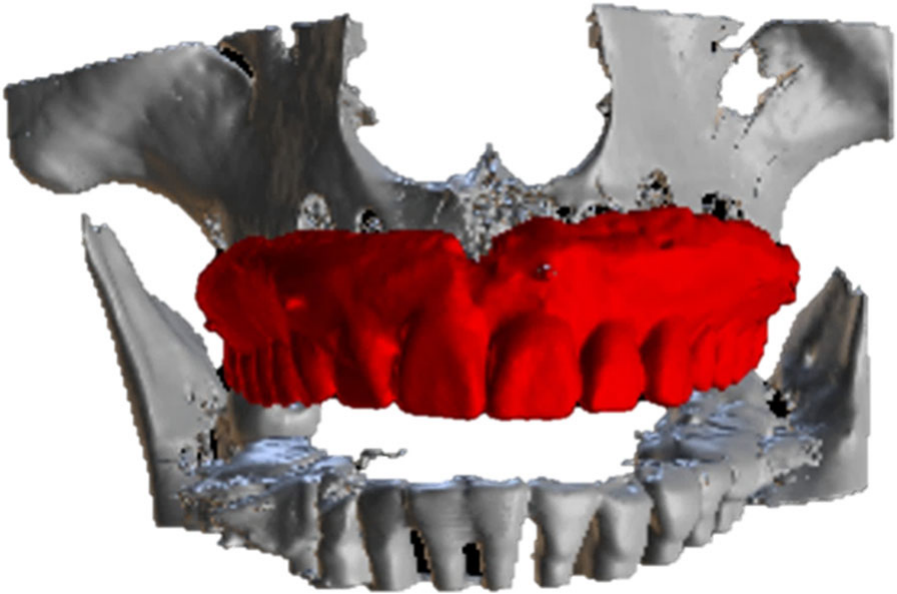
Alignment of denture scan to patient’s maxillary CBCT scan
